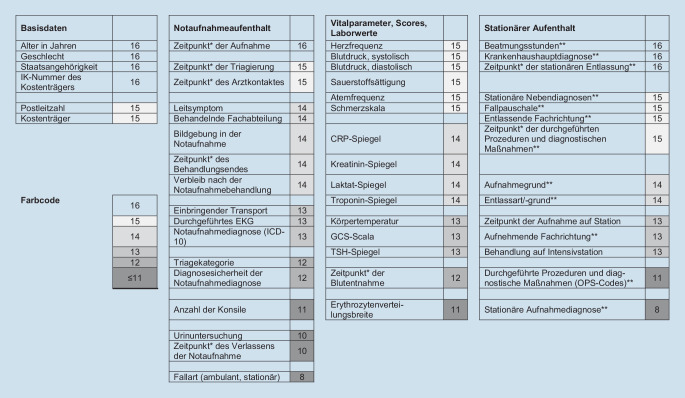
Folgende Änderungen wurden im Vergleich zu der initialen Variablenliste durchgeführt:Reduktion der Laborparameter von 36 auf die sechs relevantesten Werte, welche Rückschlüsse auf die Erkrankungsschwere zulassen bzw. Bestandteil klinischer Scores sind.Abfrage der Glascow-Coma-Scale nur als Summenscore ohne die drei Einzelkategorien.Die Abfrage nach dem Vorliegen eines Einweisungsscheines wurde gestrichen, da die elektronische Verfügbarkeit als unzureichend bewertet wurde.Diagnostische Maßnahmen im Notaufnahmekontext wurden aufgrund der unterschiedlichen Dokumentationsroutinen auf ausgewählte bildgebende Verfahren (Röntgen, Sonographie, Computertomographie, Magnetresonanztomographie), Urinuntersuchung und Elektrokardiogramm beschränkt.Reduktion auf sieben Zeitstempel (d. h. Datum und Uhrzeit), insbesondere Verzicht der Zeitstempel bei den diagnostischen Prozeduren (s. voriger Punkt).Klinische Notaufnahmediagnosen liegen nicht immer vor, da in Notaufnahmen keine direkte Pflicht zur Erhebung von kodierten Diagnosen besteht. Als Surrogat wurden daher für stationäre Fälle die Aufnahmediagnosen nach §301 Sozialgesetzbuch (SGB) V und für ambulante Fälle die Abrechnungsdiagnose(n) nach §295 SGB V ergänzt [12].Der Name der Krankenkasse (Freitext) wurde zur Validierung um das Institutskennzeichen ergänzt.Ebenfalls ergänzt wurde die pseudonymisierte Betriebsstättennummer (BSNR) der Notaufnahme gemäß kassenärztlicher Abrechnung [14], damit eine Zuordnung des Leistungserbringers über die BSNR in den KV-Daten möglich ist.
